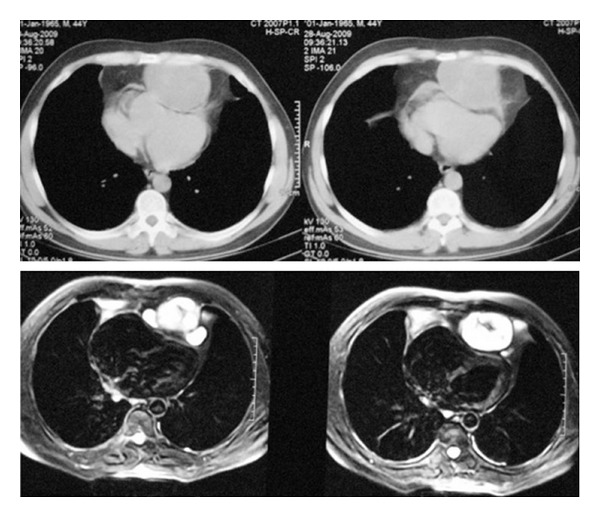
Thorax CT and MRI showing the large, encapsulated, well-defined anterior mediastinal mass lesion. The mass lesion was obviously compressing the heart.
